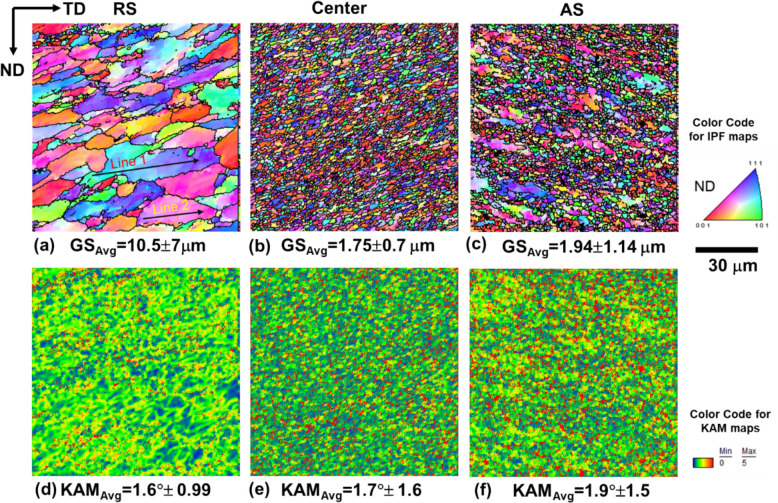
Microstructural evolution along the center line (horizontal direction) in the FSPed region: a-c ND-IPF maps and d-f KAM maps for the RS, center, and AS regions of the 600 RPM CT-FSPed sample. Note: Average grain size (GS_Avg_) was calculated considering 5° grain tolerance angle. KAM was calculated using the 3rd nearest neighbor. RS corresponds to the TMAZ, the center represents the SZ and AS denotes the interface between the SZ and TMAZ
